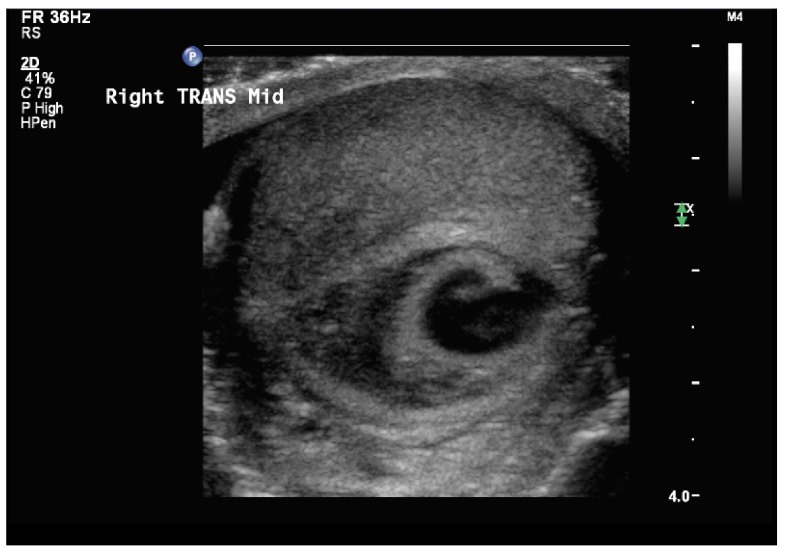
Scrotal ultrasound of the right testicle in transverse revealing a hypoechogenic lesion within the central testicle with an anechoic central core.
